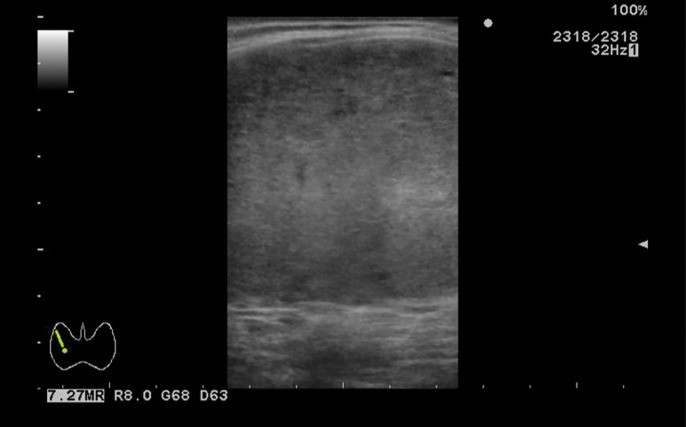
Ultrasonography. Ultrasonography revealed a hypoechoic mass measuring 100 mm in the right thyroid lobe.
